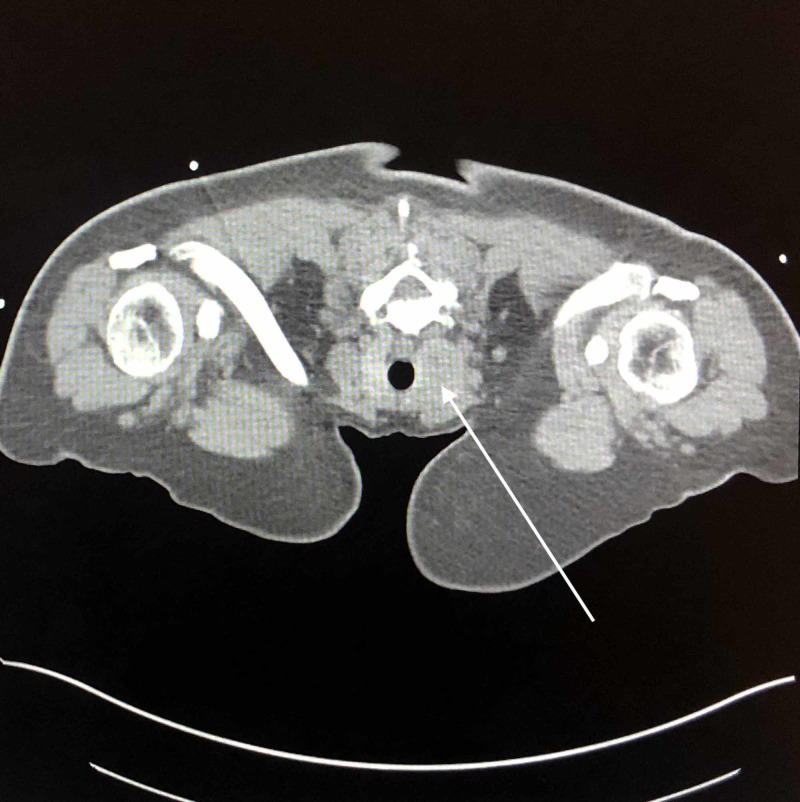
CT-guided thoracentesis and biopsy imaging for lung mass shows right thyroid nodule.
